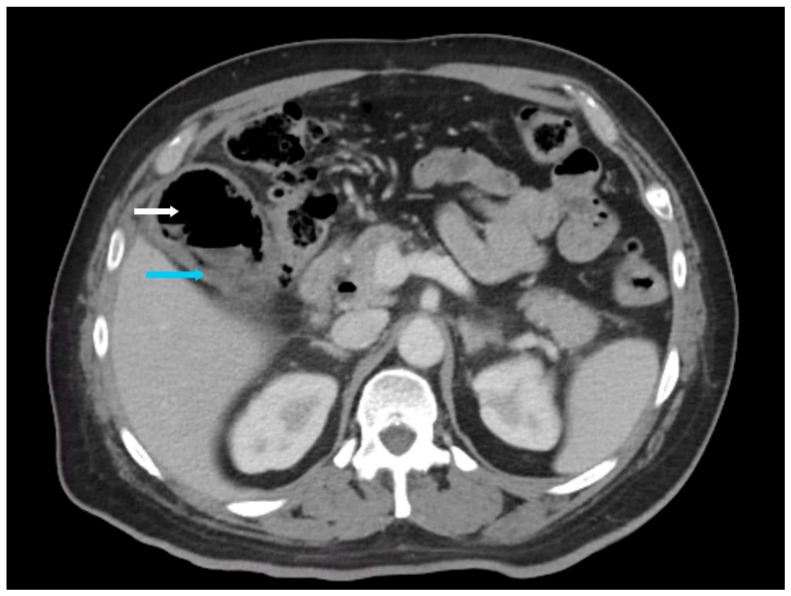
Axial contrast-enhanced computed tomography abdomen in the arterial phase (slice thickness 3 mm; soft tissue window, WW 350, WL 40) of a 39-year-old male diabetic patient with emphysematous cholecystitis presenting with abdominal pain. Extensive air in the gallbladder lumen (white arrow) and gallbladder wall edema (blue arrow) were incidentally noted.
